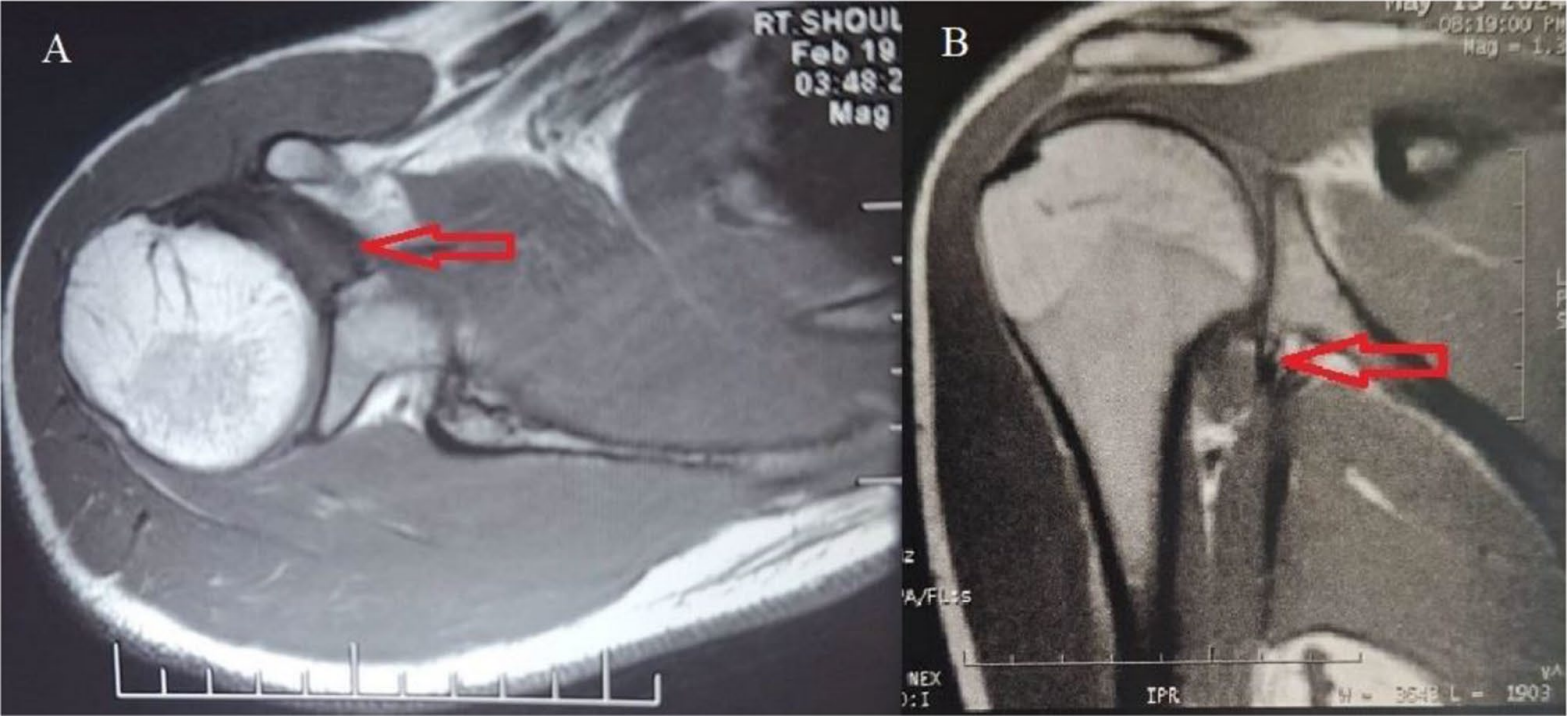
Magnetic resonance imaging showing healing of the LHB tendon to the anterior glenoid as marked by the red arrow on the (A) axial and (B) coronal slices. LHB: long head of the biceps
